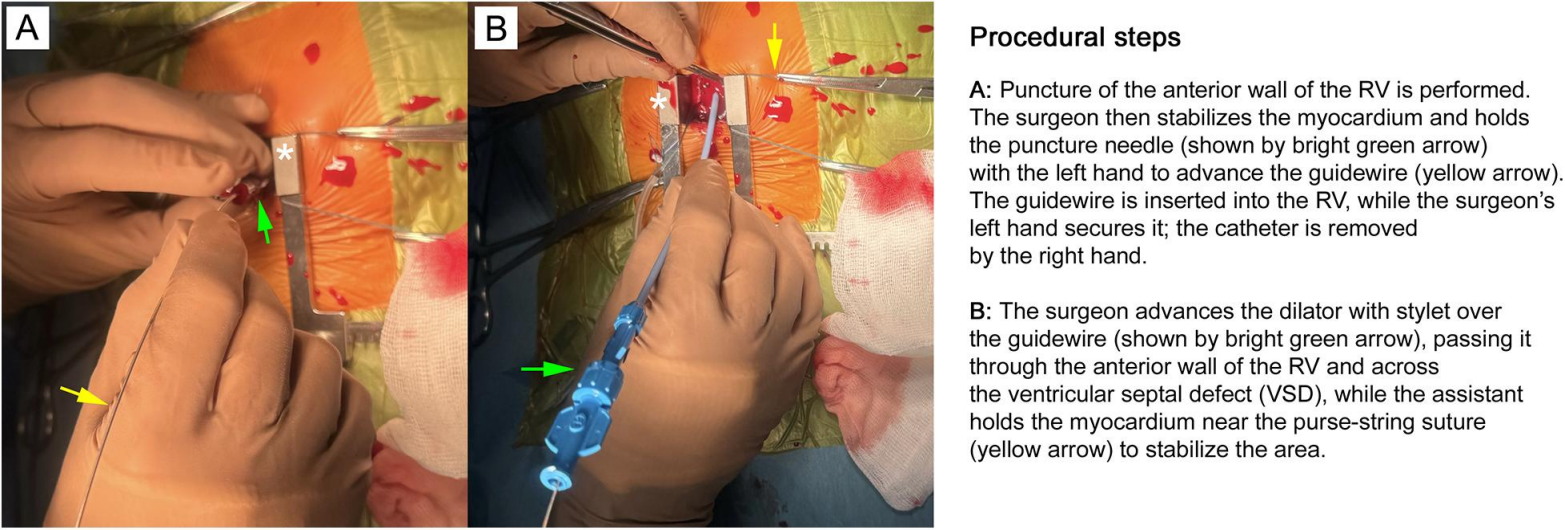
Right ventricular (RV) puncture, installation of a guidewire and dilator. (A) Puncture of the anterior wall of the RV is performed. The surgeon then stabilizes the myocardium and holds the puncture needle (shown by bright green arrow) with the left hand to advance the guidewire (yellow arrow). The guidewire is inserted into the RV, while the surgeon's left hand secures it, and the catheter is removed by the right hand. (B) The surgeon advances the dilator with stylet over the guidewire (shown by bright green arrow), passing it through the anterior wall of the RV and across the ventricular septal defect (VSD), while the assistant holds the myocardium near the purse-string suture (yellow arrow) to stabilize the area. As soon as the dilator advances over the guidewire into the left ventricular (LV) cavity, the surgeon releases the myocardium and removes the stylet along with the guidewire, while the assistant maintains the same position. This stage is considered extremely important. The surgeon's precise movements allow him to pass a rigid stylet into the defect lumen at the very first attempt without damaging the wall of the interventricular septum, where the cardiac conduction system is located near the defect crest. This helps avoid heart rhythm disturbances, which are the most serious complications with this surgical technique. The second important point is the possibility of damaging the body of the aortic valve leaflet itself with a straight rigid stylet, which can lead to its insufficiency. Therefore, each movement of the surgeon must be strictly controlled by the transesophageal echocardiographic (TEE) guidance. The retractor is shown by asterisk on both panels. The images are taken in our own surgery department, hybrid room, 11-month old patient with perimembranous VSD.
